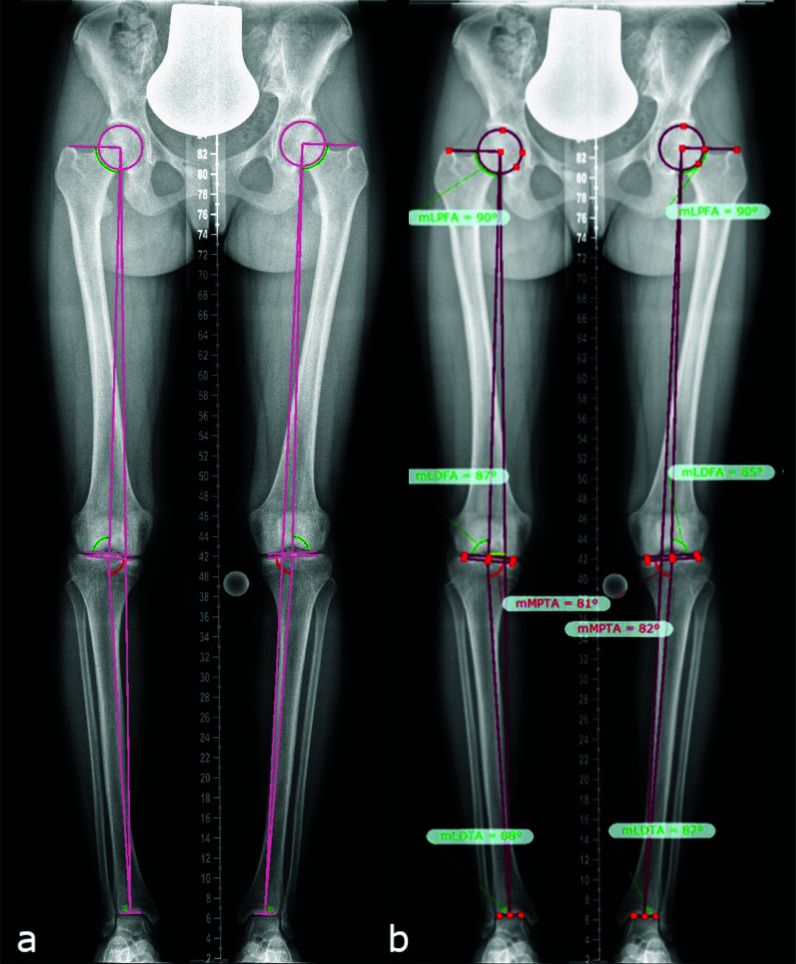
Lower limb deformity measurements on full-length anteroposterior radiograph images (hip to ankle) of both lower limbs in standing position (A), as assessed using the TraumaCAD software according to standard measurement protocols (B) (14, 15), in a patient with mild XLH (18 y/o, female, BMI of 21.6 kg/m^2^, GDI of 89.8, no surgeries, receiving conventional treatment since childhood, and mild bilateral varus deformity proximal tibia).
